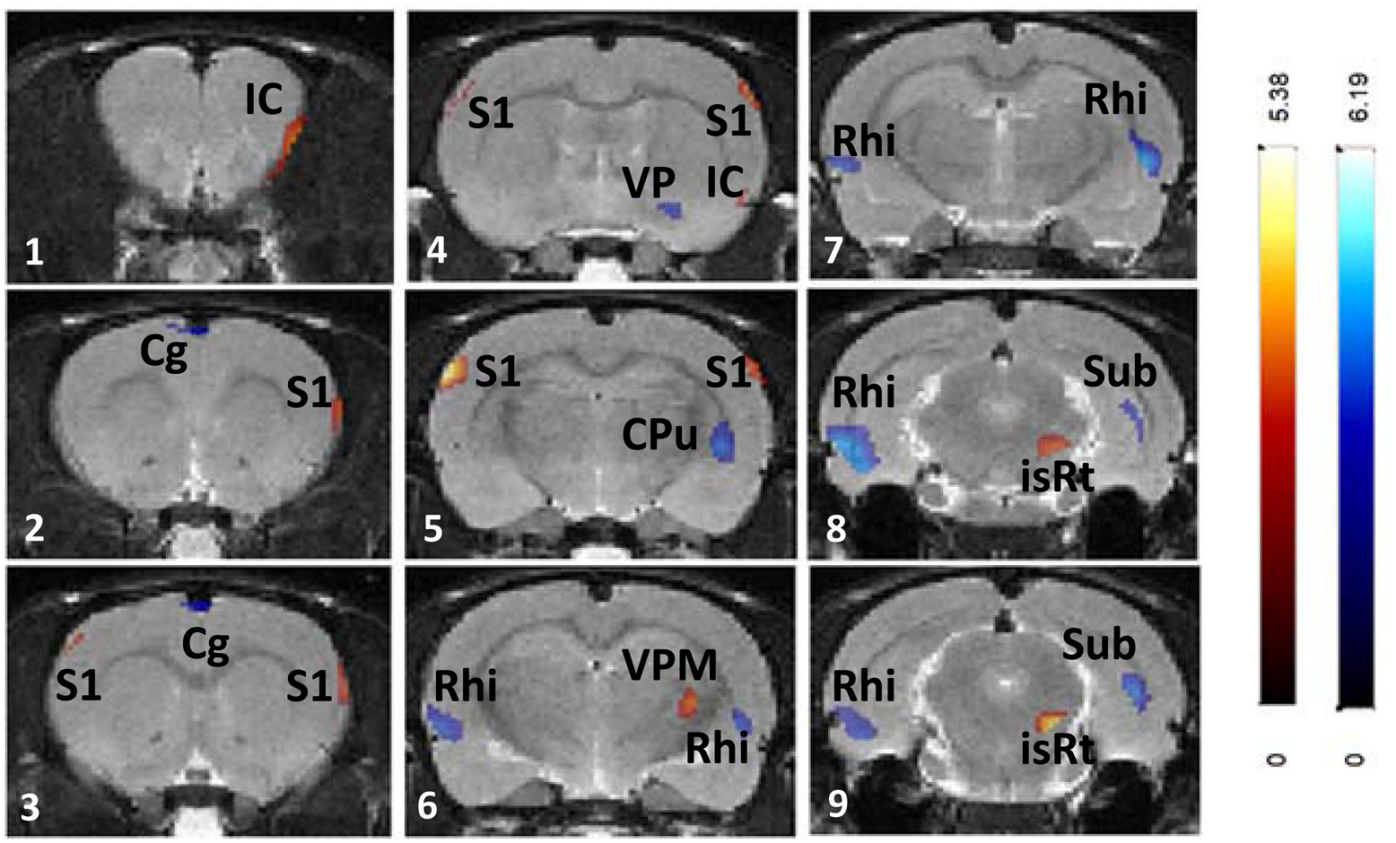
Effects of chronic LD MA (4mg/kg) treatment on regional brain glucose metabolism. Changes in regional brain glucose metabolism are superimposed on the Schweinhardt MRI template, in Paxinos space. Red: brain regions with increased glucose metabolism after 4 mg/kg MA treatment compared with vehicle treatment; Blue: brain regions with decreased glucose metabolism after 4mg/kg MA treatment compared with vehicle treatment. IC: insular cortex, Cg: cingulate cortex; S1: primary somatosensory cortex; VP: ventral pallidum; CPu: caudate-putamen (striatum); Rhi: rhinal cortices; VPM: ventral posteromedial thalamic nucleus; isRt: isthmic reticular formation; Sub: subiculum.
